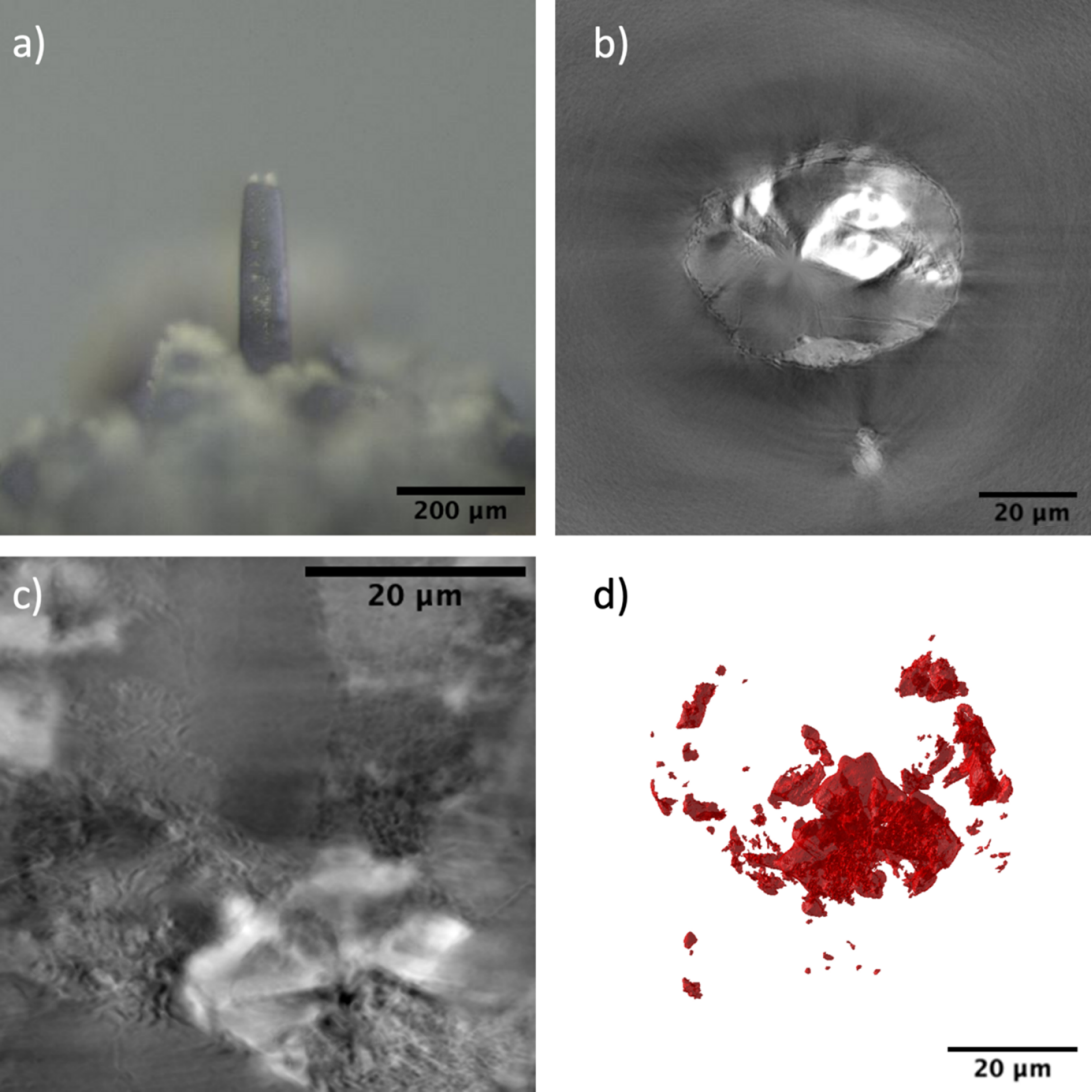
(*a*) Optical image of the basalt nano-CT pillar sample. (*b*) Vertical and (*c*) horizontal slice of the basalt nano-CT reconstructed data. (*d*) 3D visualization of the bright particles.
